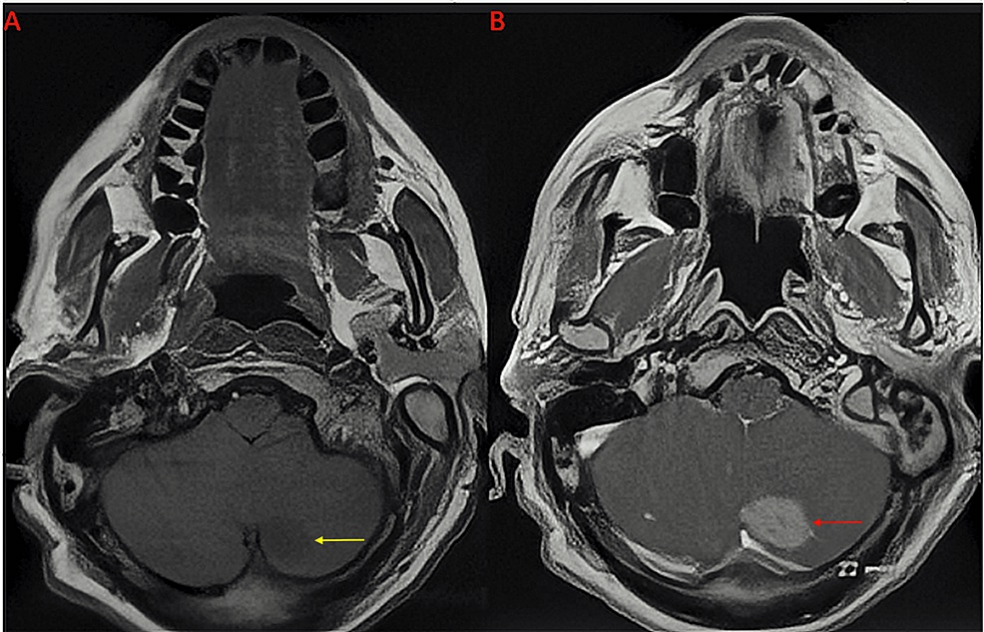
Preoperative MRI of the brain with and without contrast in the axial plane Precontrast (A) and postcontrast (B). The lesion is hypointense on precontrast T1-weighted sequence (yellow arrow) and shows diffuse postcontrast homogenous enhancement (red arrow) MRI: magnetic resonance imaging
